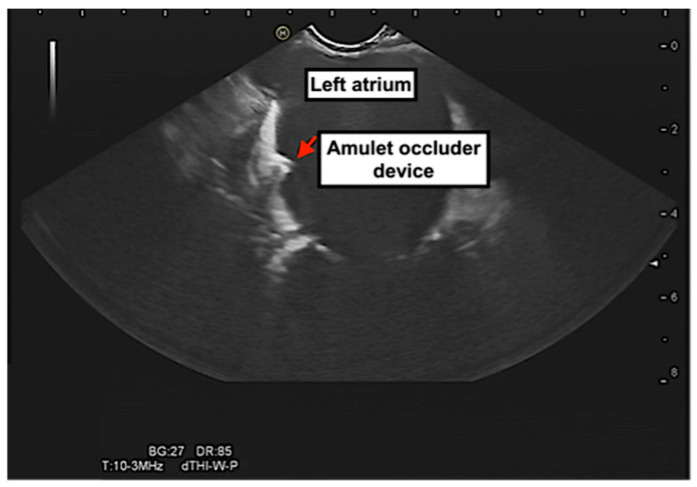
Ultrasonographic ultrasound demonstrated with another view the morphology of amulet occluder device of left atrial appendage without leak, thrombus or vegetation.
